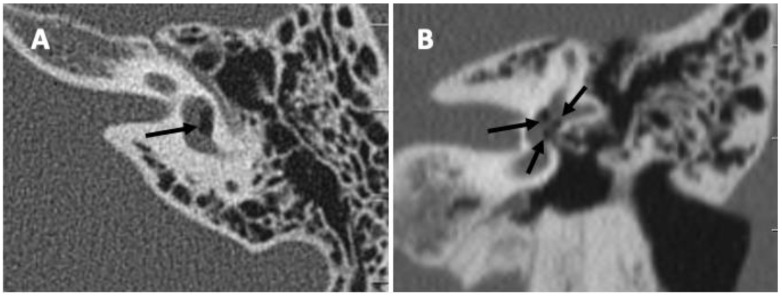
CT scan showing the pneumolabyrinth (arrows) on axial (A) and coronal (B) cuts.
